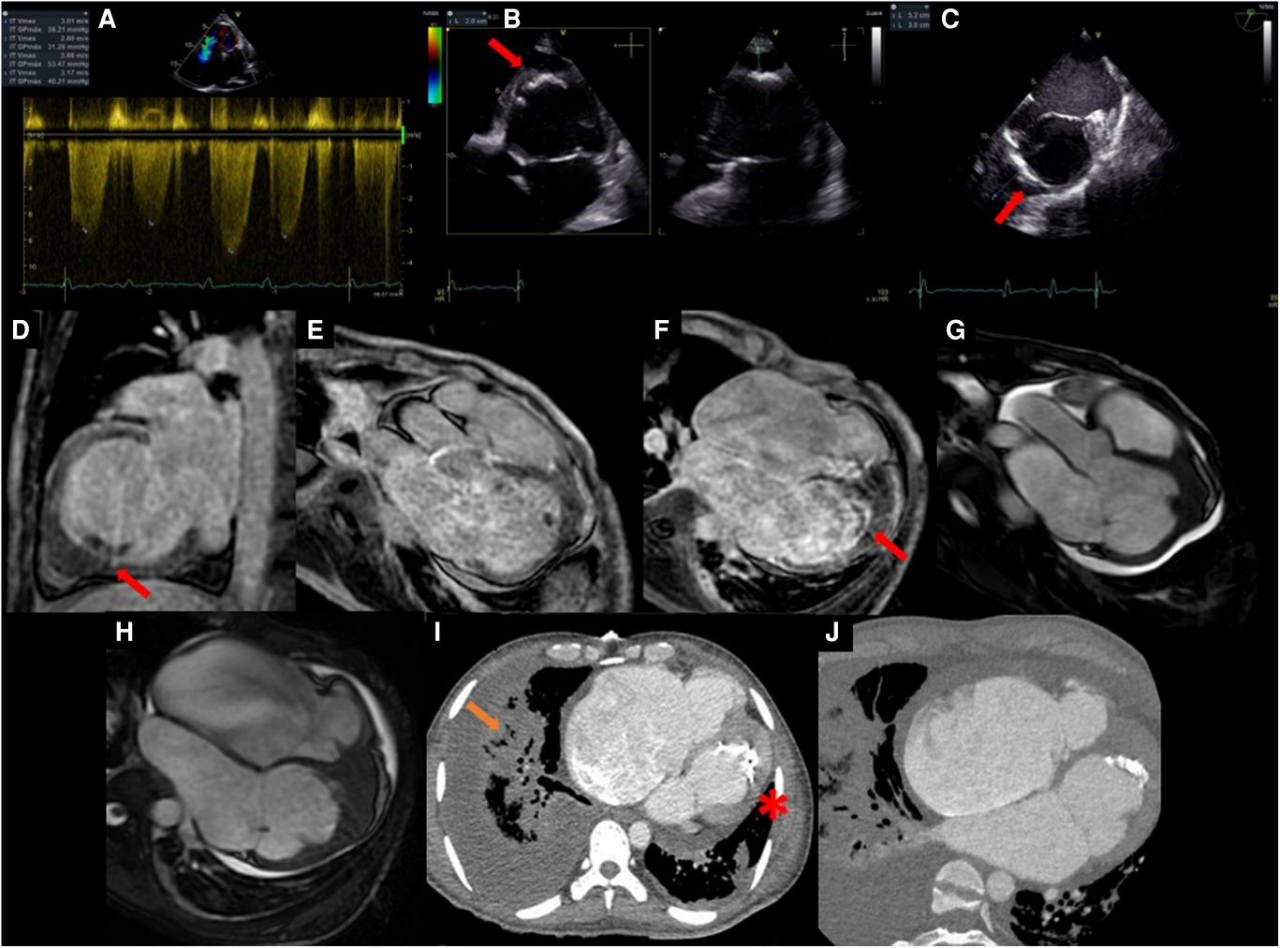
Case 2 images. (*A*) Continuous Doppler at the level of the tricuspid valve with severe regurgitation (peak velocity of 3 m/s) and septal e′ wave tissue Doppler 6 cm/s (not shown). (*B*) Echo apical four-chamber view focused on the left ventricle, and (*C*) transoesophageal echocardiography mid-oesophageal view at 60° shows calcified endocardium superimposed on apical left ventricular hypertrophy with a maximum thickness of 15 mm (red arrows). (*D–F*) Late gadolinium enhancement sequences showing the ‘double V sign’ (red arrows) with endocardial enhancement and superimposed apical thrombus. (*G*, *H*) Steady-state free precession sequence in three-chamber and four-chamber views, respectively, with significant bi-atrial dilatation and biventricular apical obliteration during diastole. (*I*, *J*) Cardiac computed tomography showing large endocardial apical calcification (asterisk) and apical obliteration with hypoattenuated image. Note the pulmonary congestion with air bronchograms (orange arrow).
